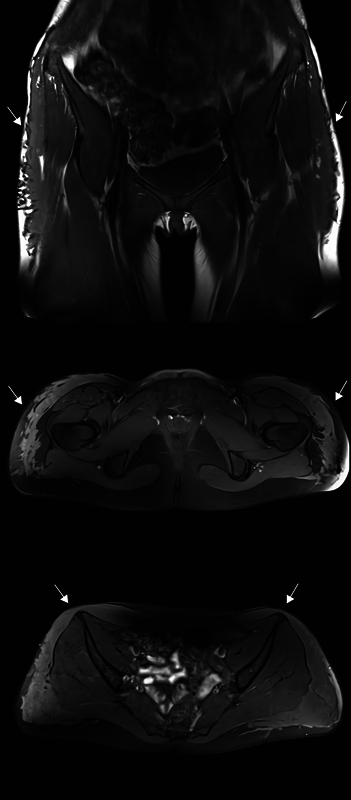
Magnetic resonance imaging (MRI) showing migration of the filler to the proximal part of both thighs and inguinal regions highlighted by arrows.
